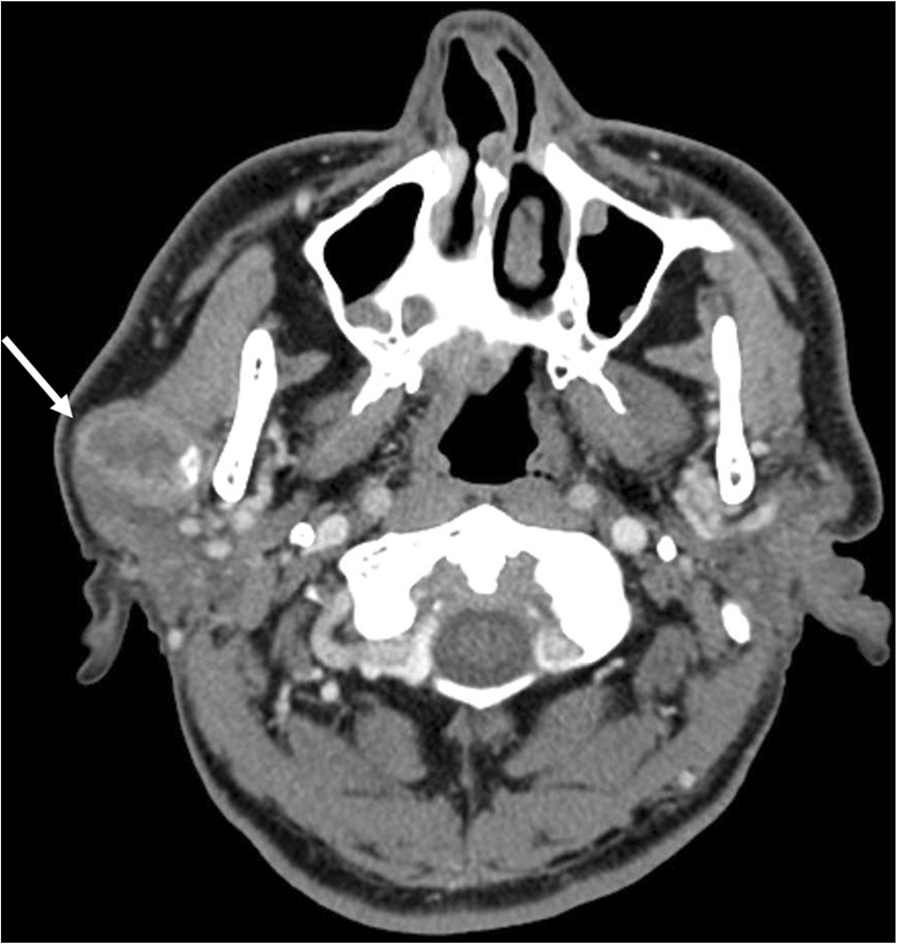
A heterogeneous enhancing solid lesion with calcification (arrow) was identified in the superficial lobe of right parotid gland on neck CT
